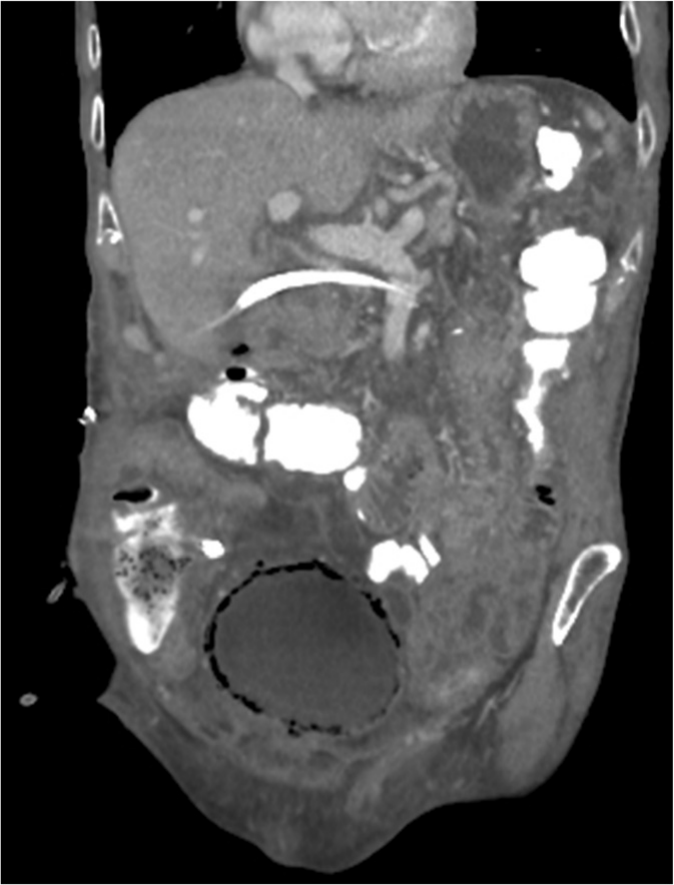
Computed tomography. Coronal view revealing emphysematous changes in the bladder wall.
